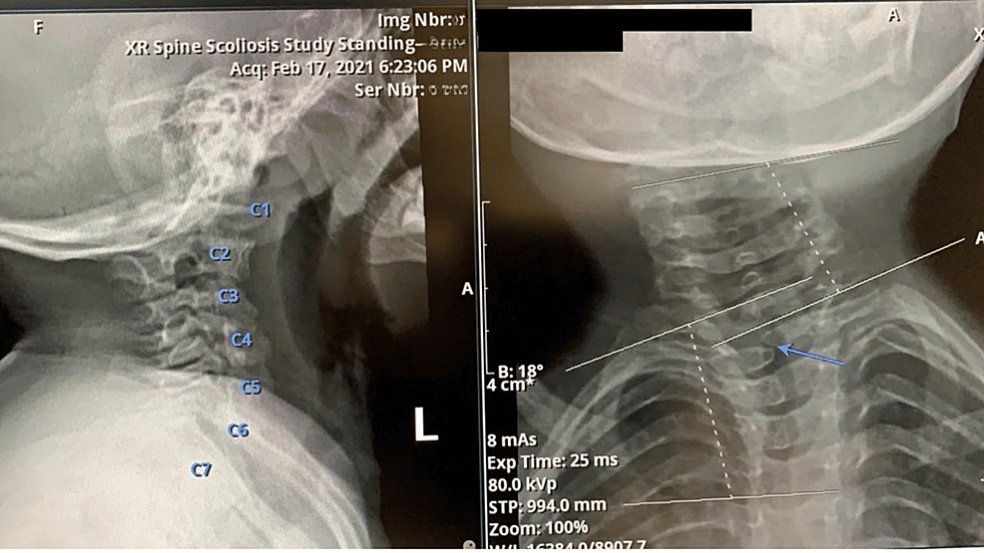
A closer view of the X-ray images, showing the C2 and C3 butterfly-shaped fusion
